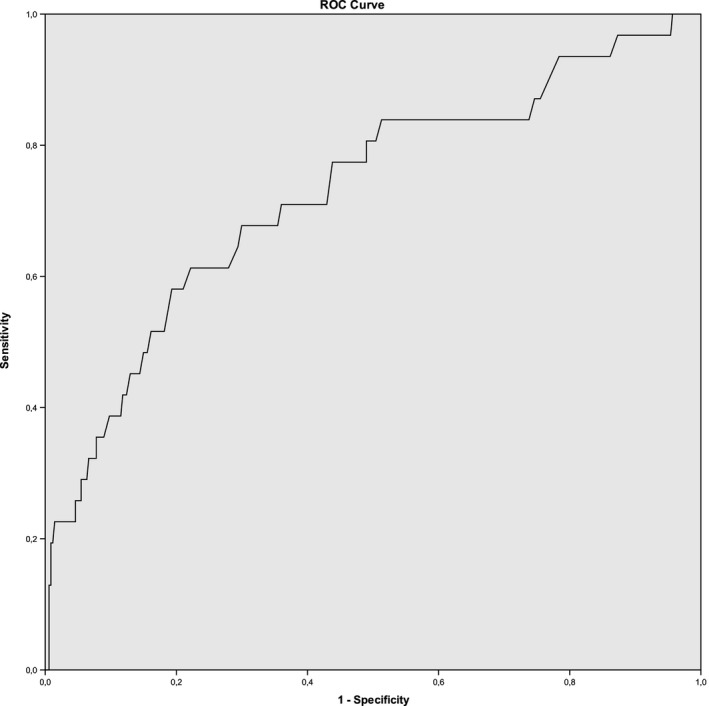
Receiver operating characteristic curve. Detection of significant vertebral artery stenosis (≥50%) on CTA by DUS based on peak systolic velocities (PSV) for the V1 segment of the vertebral artery
